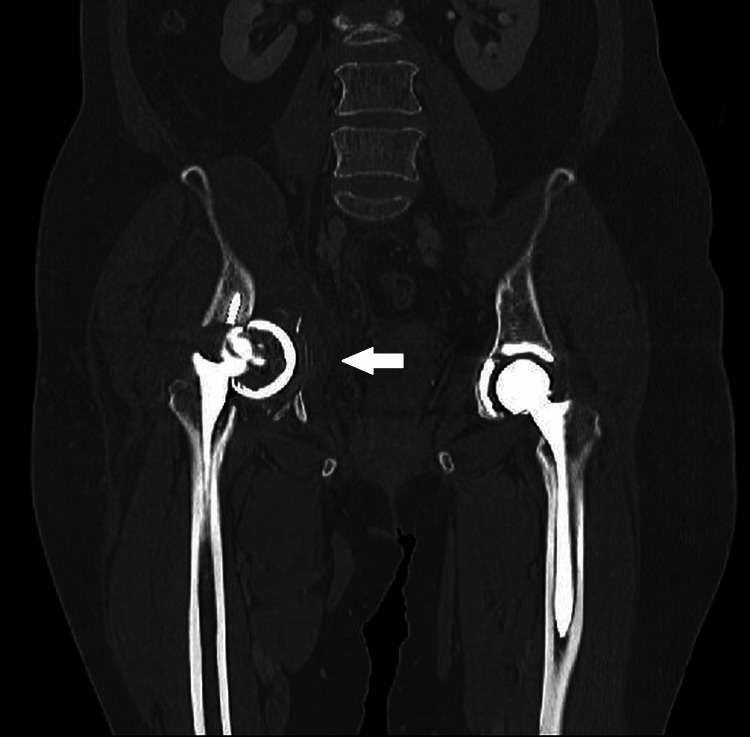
Pelvic coronal CT image after the secondary fall. There was increased displacement at the fracture site (arrow), accompanied by central dislocation. The acetabular cup was dislodged, and the screws were fractured.
